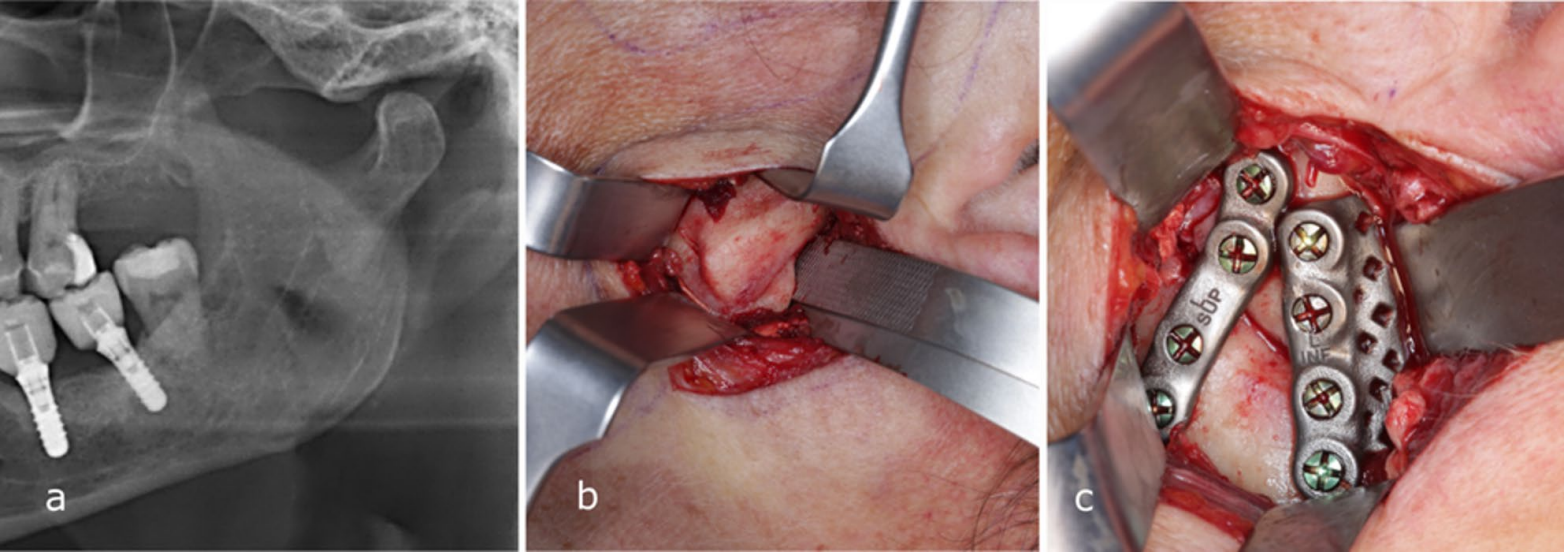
a Panoramic radiograph of patient 7 showing a laterally displaced, healed condyle following a condylar base fracture. b Intraoperative photograph of the laterally displaced condyle. c Intraoperative photograph after osteotomy, repositioning, and fixation with two patient-specific plates
